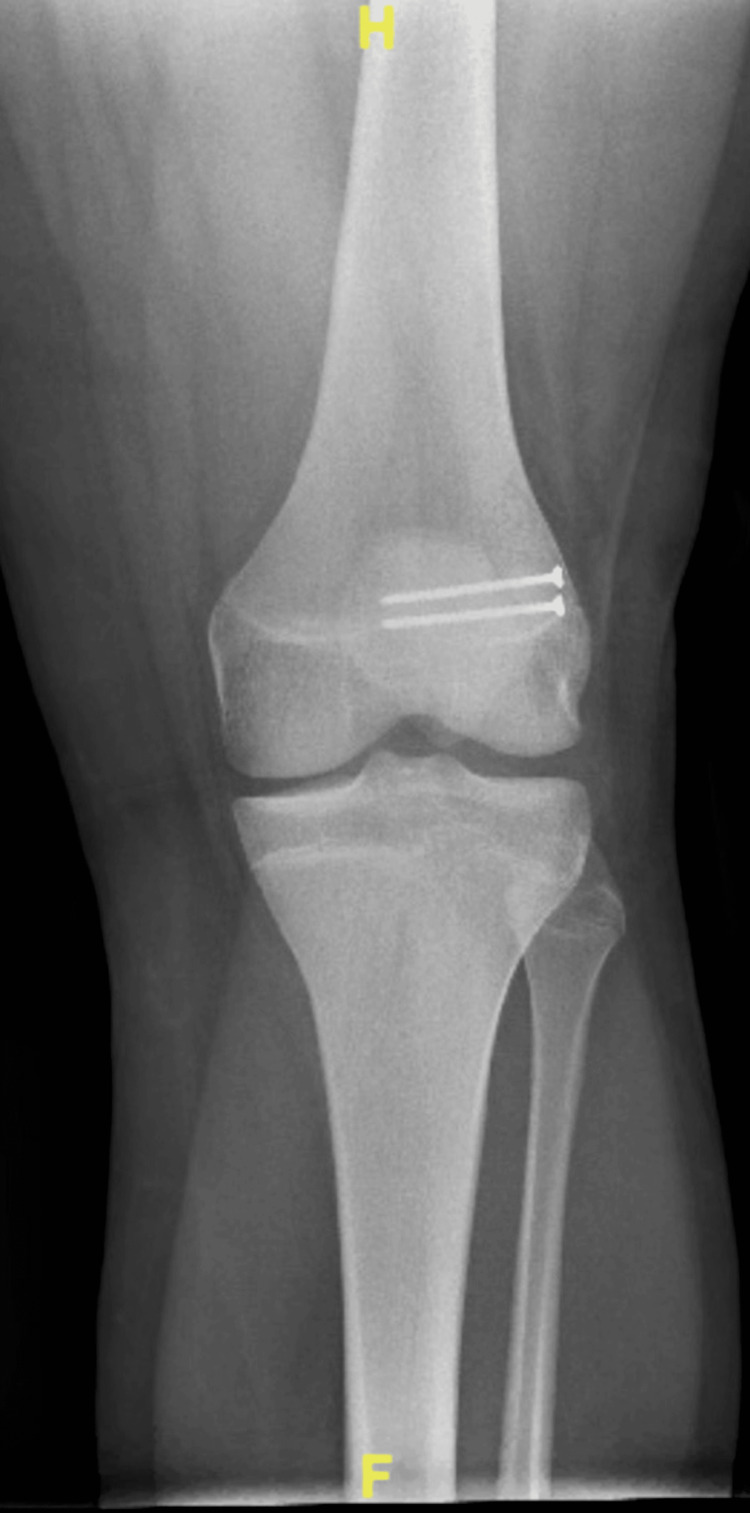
Postoperative X-ray L knee PA view PA: Posteroanterior.
